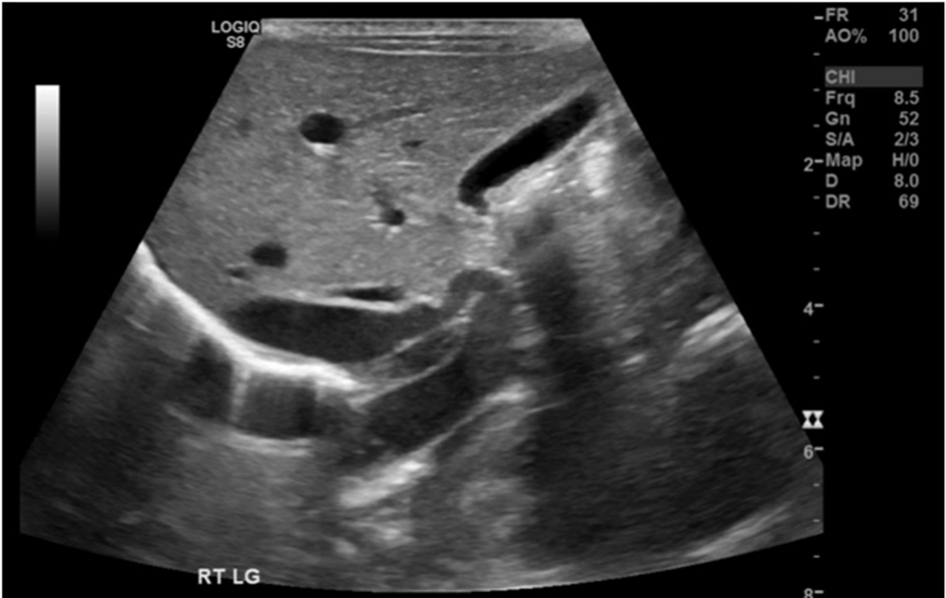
Primary diagnostic ultrasound revealing cIAPF.
